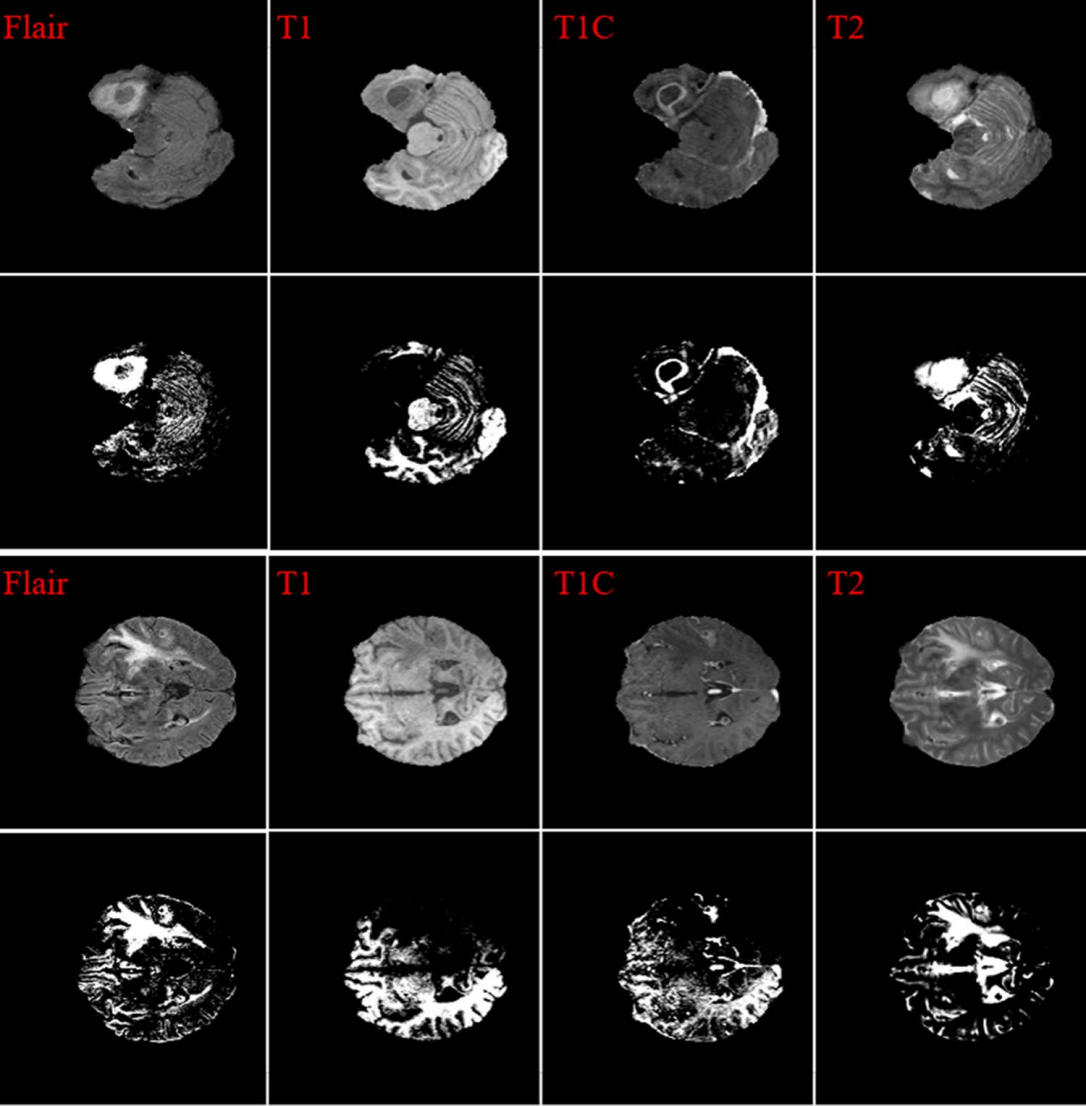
Two sets of four MRI modalities and their corresponding Z-Score normalization.
